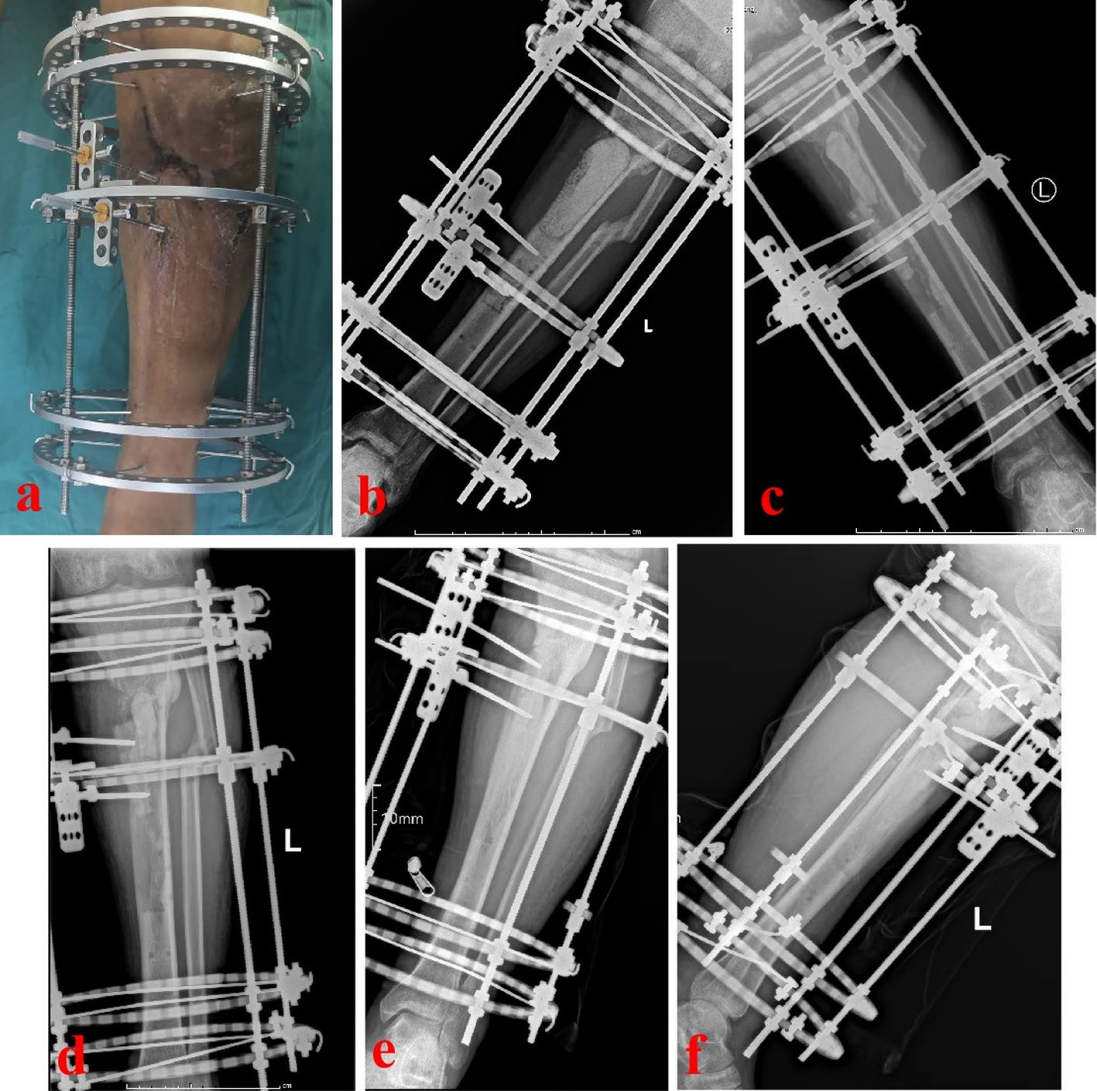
The patient was treated by the semi-focal bone transport technique. (a) Appearance of the injured limb during the semi-focal bone transport process; (b–d) X-ray films of the semi-focal bone transport process; (e–f) X-ray films showed that the consolidation of new callus was good.
